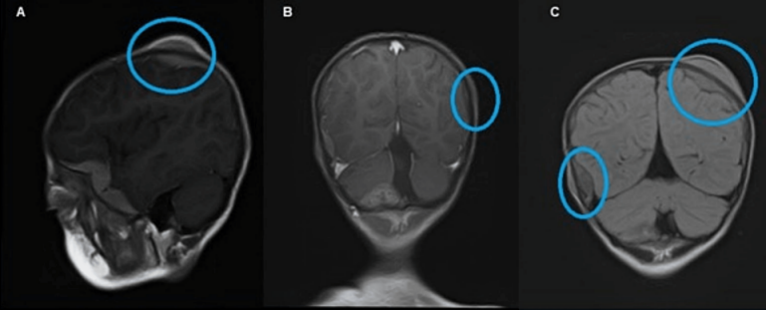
MRI showing multifocal scalp-based lesions with enhancing extradural soft tissue component seen along the left greater wing of sphenoid, bifrontal, and left parietal region producing bulge and mild leptomeningeal enhancement. Sagittal MRI (A), post-gadolinium coronal MRI (B), and FLAIR (C) show multifocal scalp-based lesions with enhancing extradural soft tissue components seen along the left greater wing of sphenoid, left high parietal, and left occipital region.
